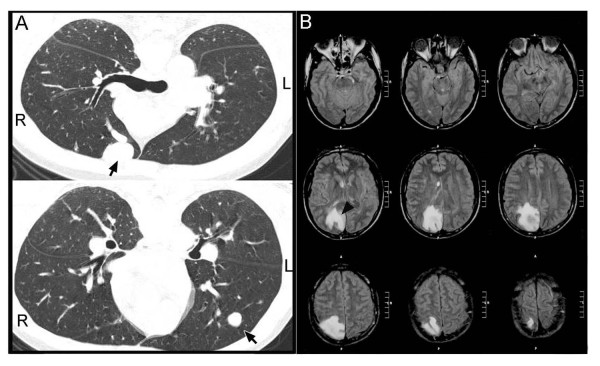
Clinical features of an HHT patient (I-2). PAVM (arrows) and cerebral abscess (arrow head) are detected by chest computed tomography (CT) (A) and brain CT (B), respectively.
